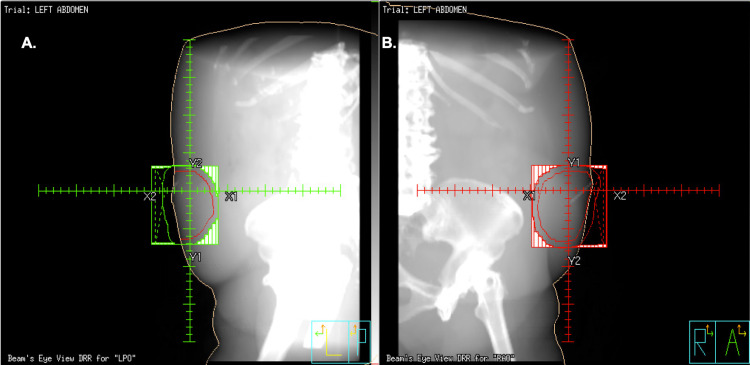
Digitally reconstructed radiograph (DRR) images Digitally reconstructed radiograph (DRR) images showing the beam’s eye view of the left posterior oblique (LPO; A) and right anterior oblique (RPO; B) fields, shaped with multi-leaf collimators around the planning target volume (marked with a red contour along the skin surface). Wedges were utilized to improve homogeneity. Daily tissue-equivalent bolus applications were performed to ensure prescription dose at surface.
